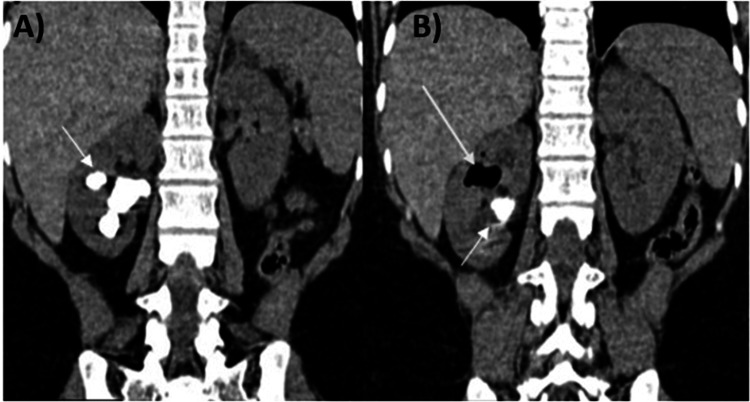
Coronal plane CT images Staghorn calculus in the right renal pelvis (A and B, short arrows). Multiple air foci in the upper and interpolar calyces (B, long arrow).
